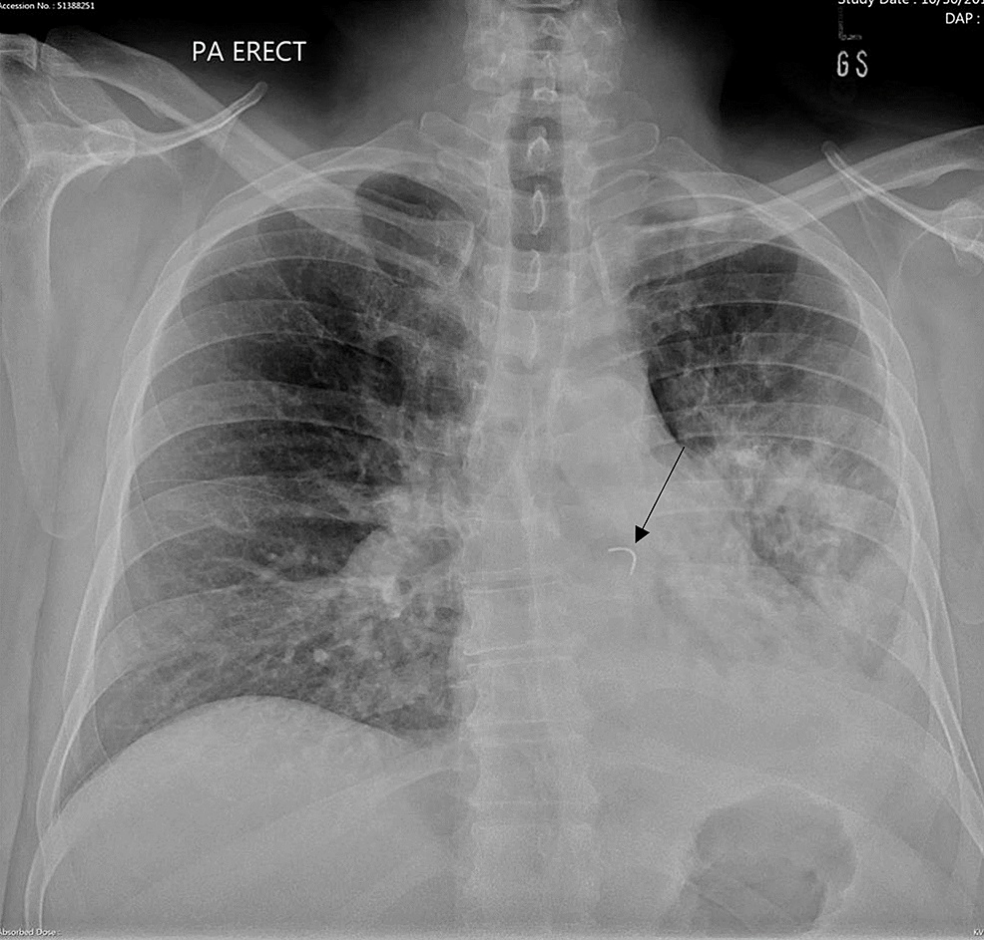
PA view of the chest X-ray chest. A curved radio-opaque density is seen projecting over the left lower lobe bronchus (black arrow). Inhomogeneous shadowing in the left mid and lower lung zone with air bronchogram representing consolidation and obscured left hemidiaphragm and left costophrenic angle suggesting mild left pleural effusion. PA: posteroanterior
